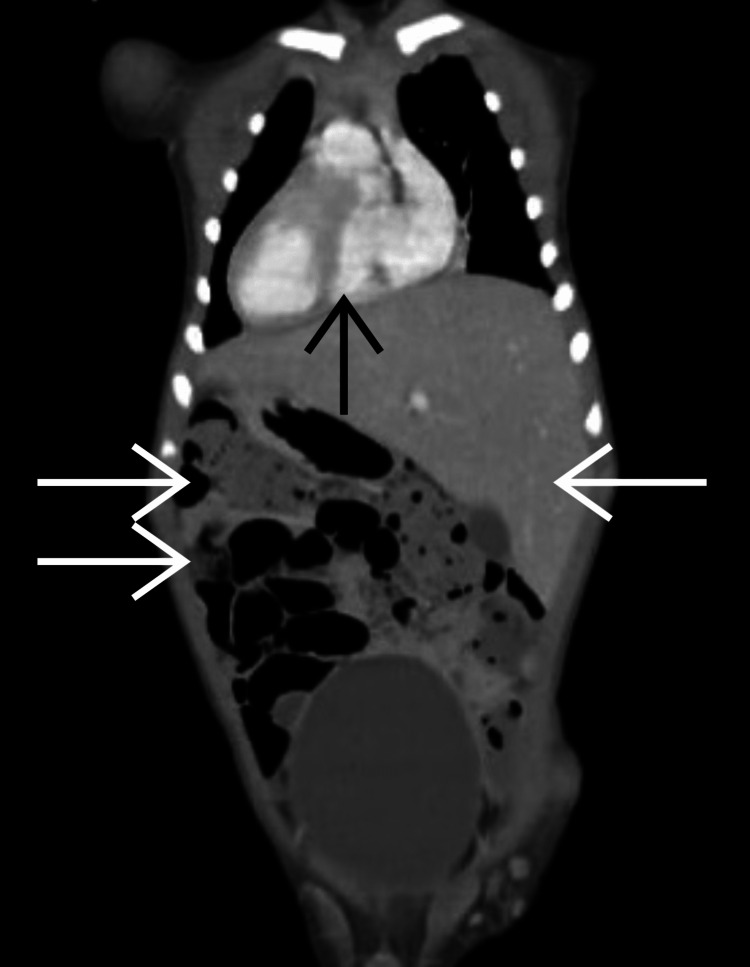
Coronal section of the abdomen and chest CT exhibiting situs inversus totalis (dextrocardia demonstrated by a black arrow and peritoneal organ inversion demonstrated by white arrows)
